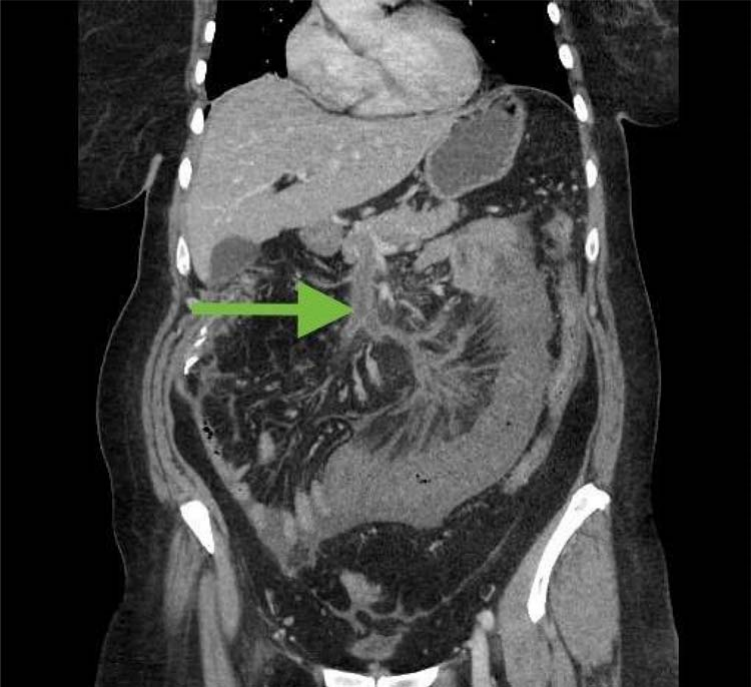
CT abdomen/pelvis 2 weeks after initial surgery demonstrating superior mesenteric vein thrombosis (arrow) and a loop of thickened small bowel consistent with ischemia.
